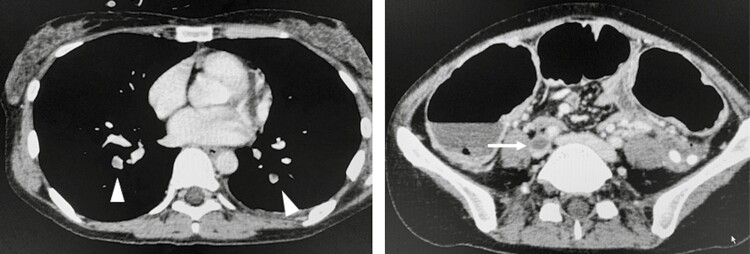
A 39-year-old woman who underwent emergency surgery for toxic megacolon. Contrast-enhanced computed tomography revealed pulmonary embolism (enhanced CT diagnosed pulmonary embolisms (denoted by △) and inferior vena cava thrombosis (denoted by →).
